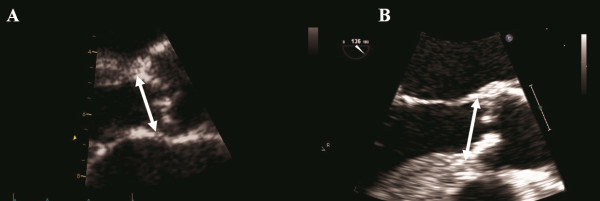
A and B. Aortic annulus measurements performed by transthoracic and transesophageal echocardiography. Aortic annulus dimension is indicated by the arrow.
